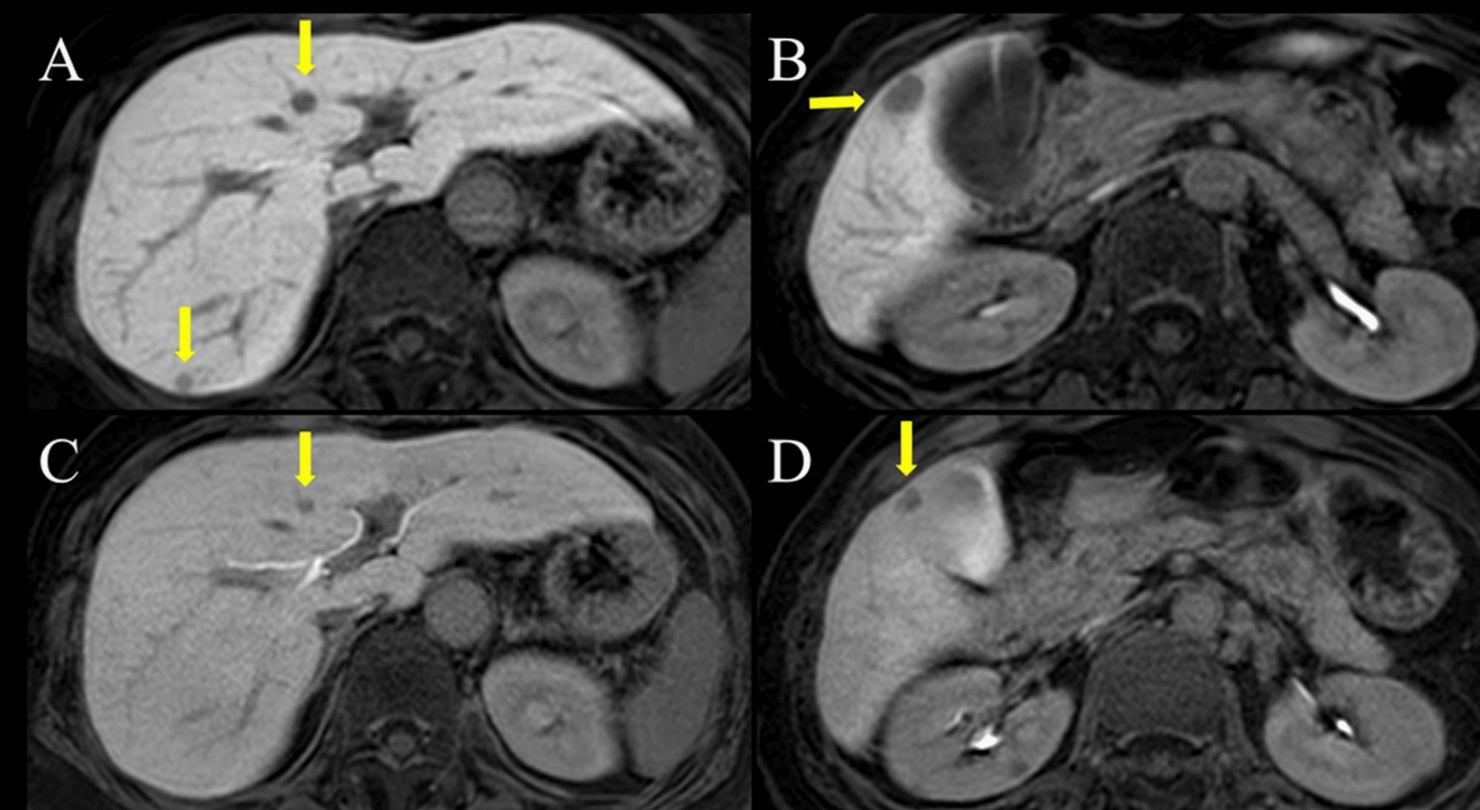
Abdominal MRI A, B: MRI showing liver tumors in segments IV, V, and VII before NAC (arrow); C, D: The tumors show a partial response after four courses of CAPEOX with bevacizumab (arrow). The tumor in segment VII was not clearly visible on the MRI slice. MRI: magnetic resonance imaging, CAPEOX: capecitabine and oxaliplatin
